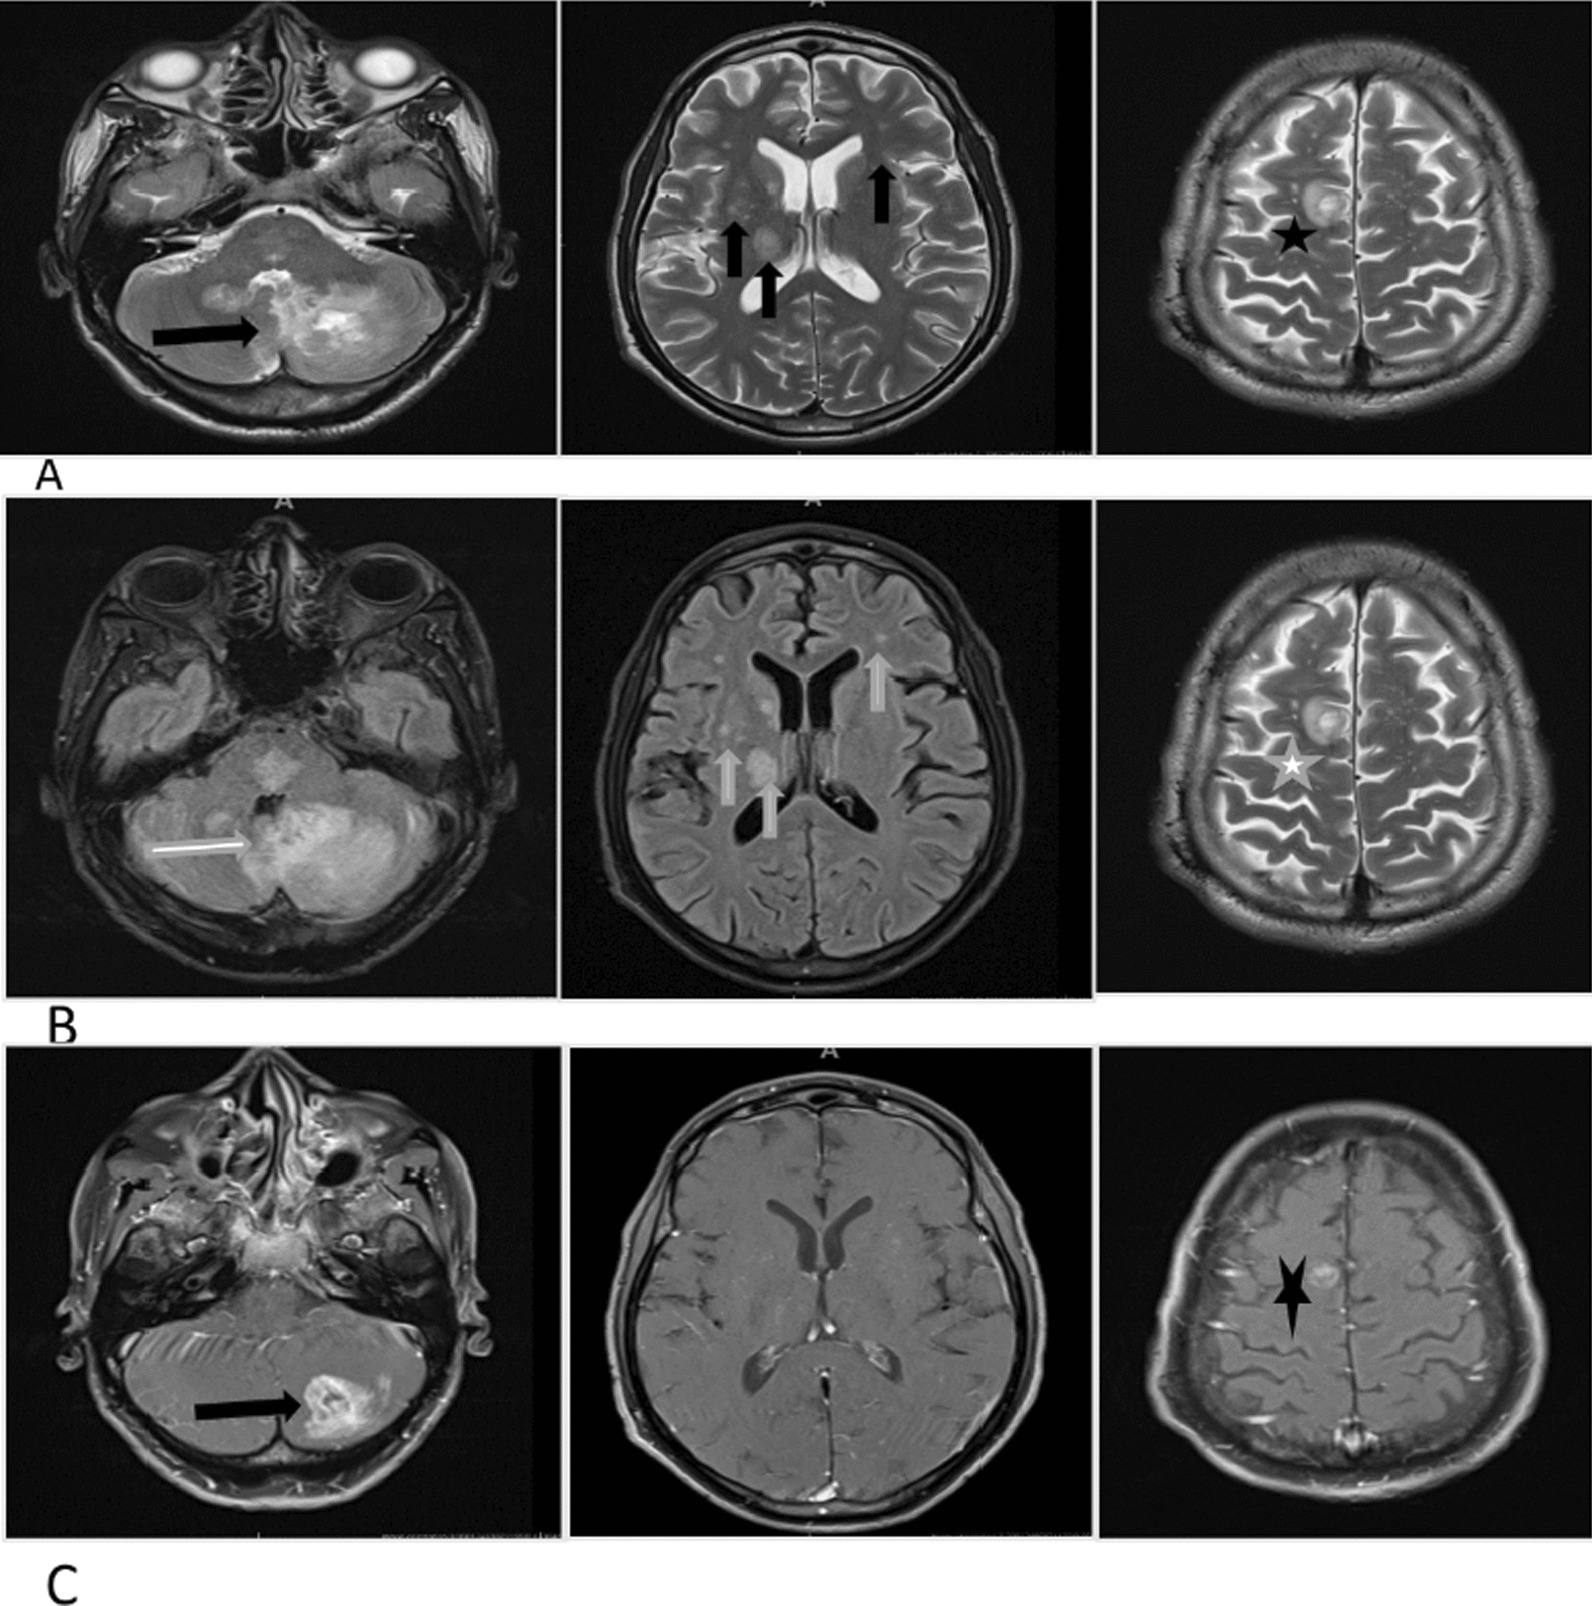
Pre-and post-contrast brain magnetic resonance imaging of case 1. Panel A (T2 weighted axial images): multiple bilateral hyperintensities demonstrated in the white and gray matter, with involvement of bilateral white mater lesions, the basal ganglia, thalami (black vertical arrows). A round subcortical right high parietal lesion with central hyperintensity and hypointense rim (black star). There is also a larger poorly defined lesion in the left cerebellum, with heterogenous signal intensity (black horizontal arrow). Panel B (Fluid attenuated inversion recovery axial images): multiple bilateral hyperintensities demonstrated in the white and gray matter, with involvement of bilateral white mater lesions, the basal ganglia, thalami (white vertical lines). A round subcortical right high parietal lesion with central hyperintensity and hypointense rim (white star). There is also a larger poorly defined lesion in the left cerebellum, with heterogenous signal intensity (white horizontal arrow). Panel C (T1 post-contrast axial images): an irregular peripheral enhancement of a subcortical right high parietal lesion (black star) and a prominent heterogenous enhancement with central non-enhancing foci of a lesion in the left cerebellum (black horizontal arrow)
